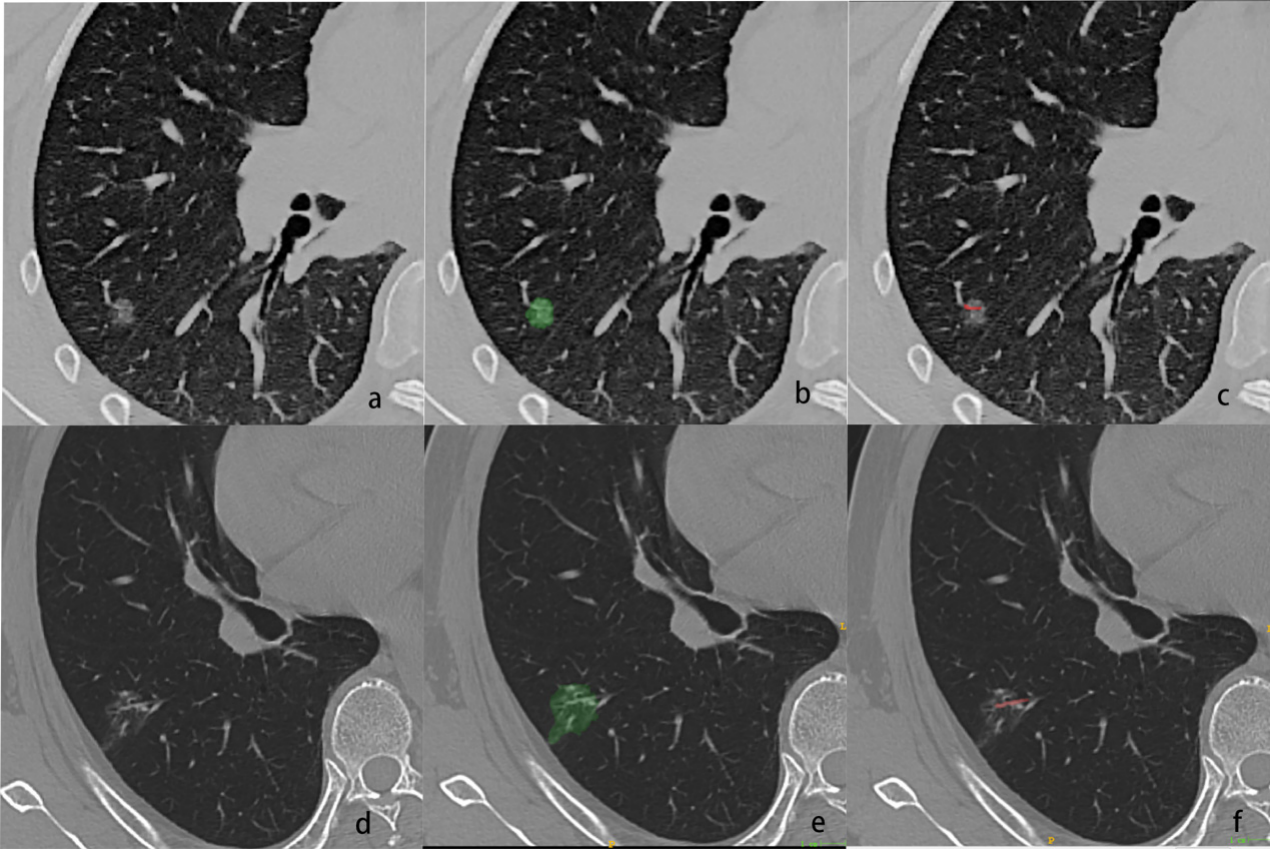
A 46-year-old woman with AIS presented as a pGGN. The nodule had a long diameter of approximately 0.7 cm and a short diameter of approximately 0.5 cm, with an average CT value of −524 HU. (a) CT showing the nodule with a regular shape, without lobulation, spiculation, or pleural retraction. (b) The ROI of the largest cross-section of the nodule. (c) The ROI of the internal vascular structures within the nodule. (d–f) A 68-year-old man with MIA presented as an mGGN. The nodule had a long diameter of approximately 1.4 cm and a short diameter of approximately 0.7 cm, with an average CT value of −422 HU. (d) CT showing the nodule with an irregular shape, lobulation, and pleural retraction but no spiculation. (e) The ROI of the largest cross-section of the nodule. (f) The ROI of the internal vascular structures within the nodule.
